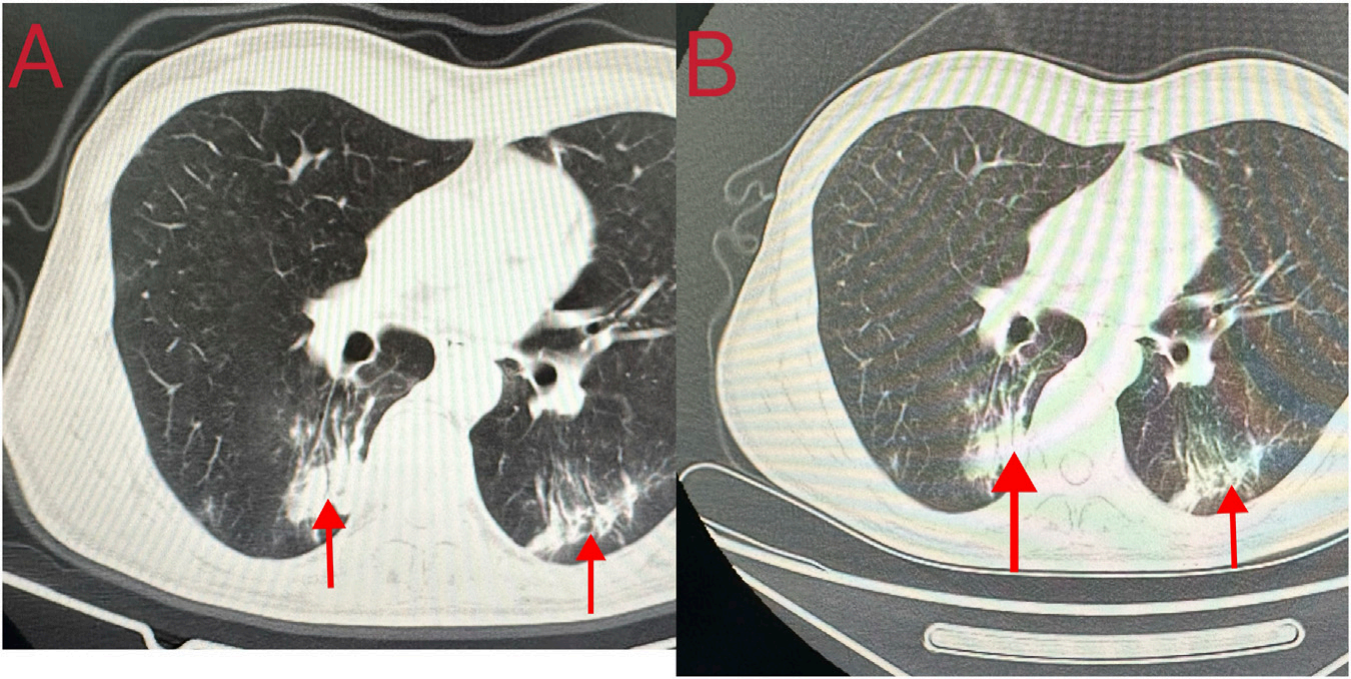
Chest computed tomography findings of the patient during the second adimission. (A, B) A high-density shadow in the lungs.
